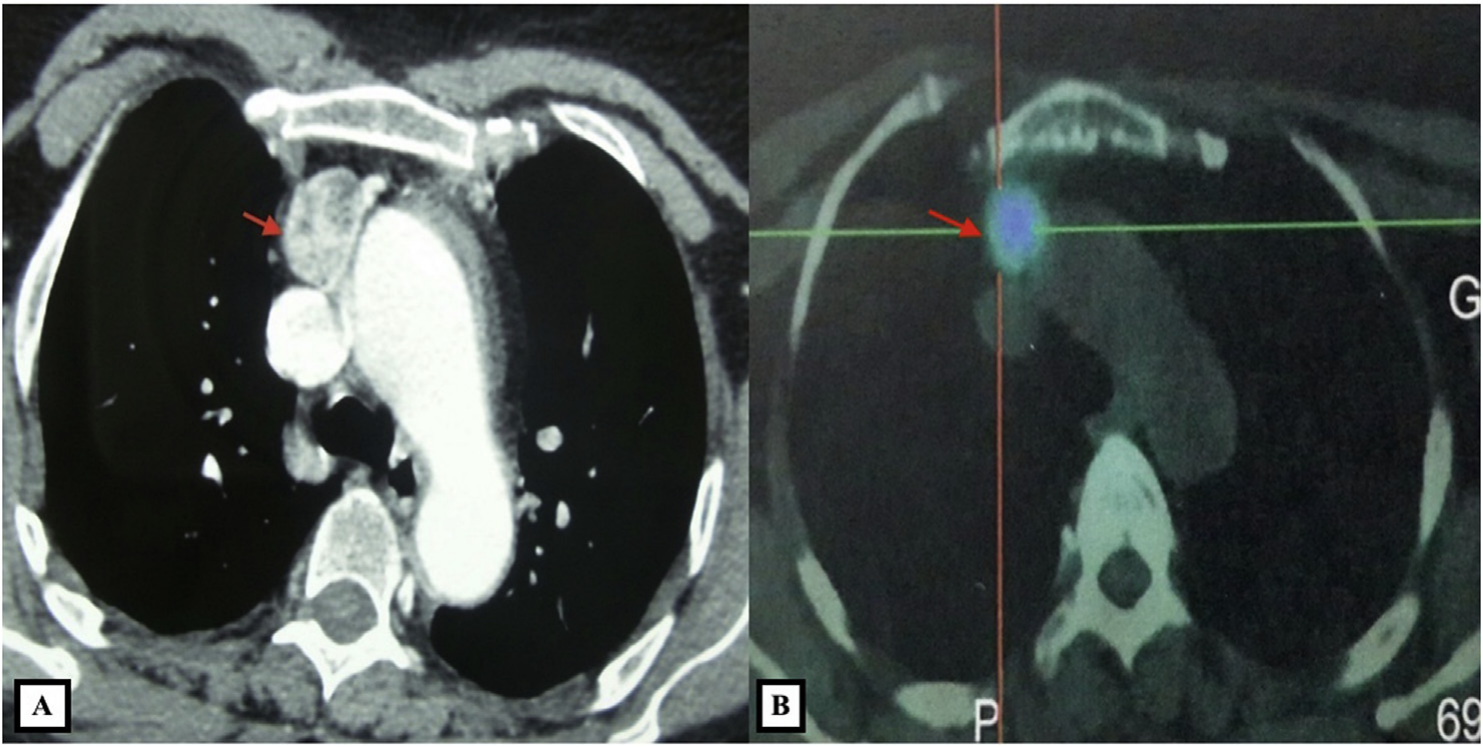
A: Thoracic CT-scan showing anterior mediastinal tissular mass with enhancement after contrast injection. B: Sestamibi-scan (99 m Tc – MIBI) showing intense uptake by the tissular mass.
